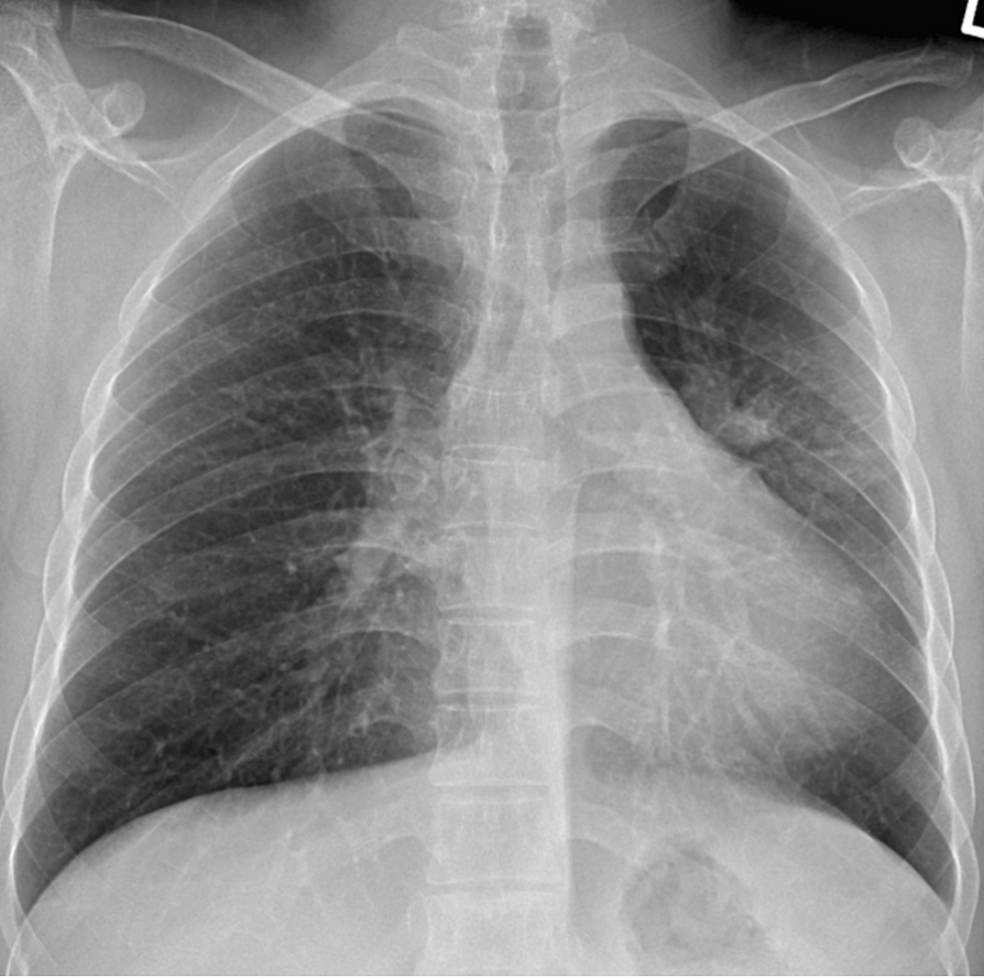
Chest X-ray of the LUL opacities showing no change (February 15, 2012) LUL: left upper lobe
